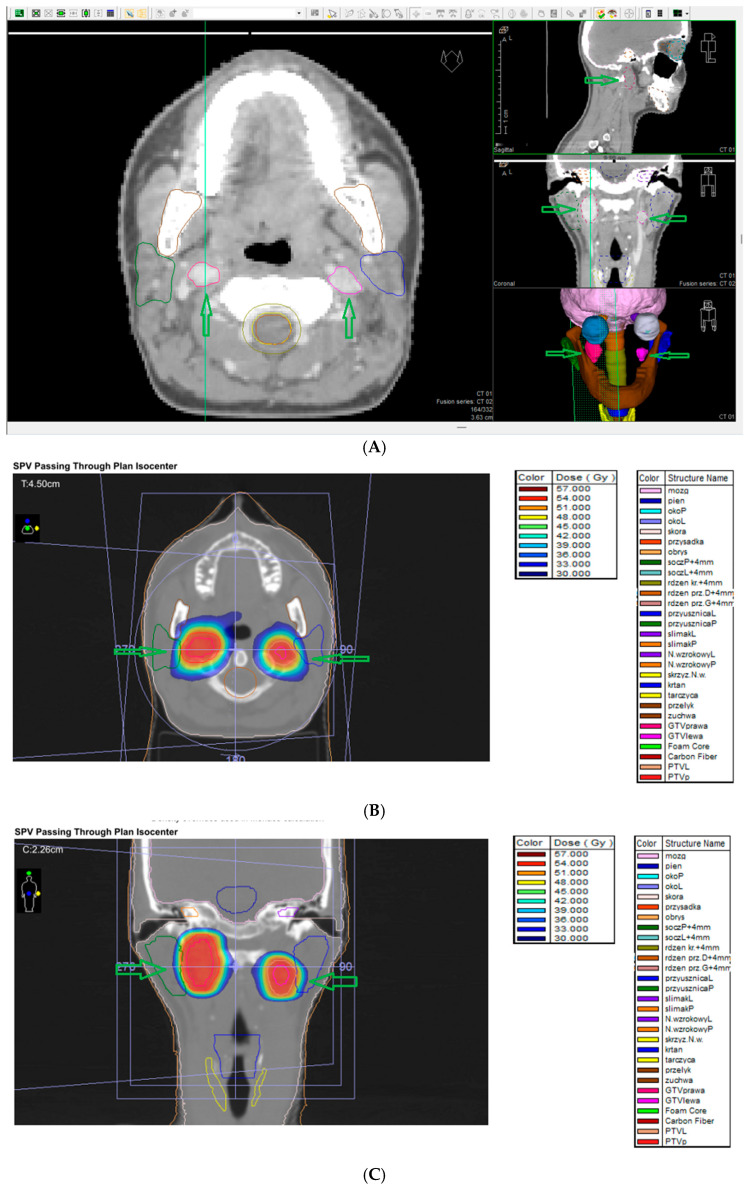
(A) Conventional radiotherapy for bilateral CBT in a 31-year-old white female patient with familial PPGL1 syndrome—SDHD C11X. CT for treatment planning; (B) conventional radiotherapy for bilateral CBT in a 31-year-old white female patient with familial PPGL1 syndrome—SDHD C11X. Dose distribution shown on the CT scan; and (C) conventional radiotherapy for bilateral CBT in a 31-year-old white female patient with familial PPGL1 syndrome—SDHD C11X. Dose distribution shown on the CT scan.
